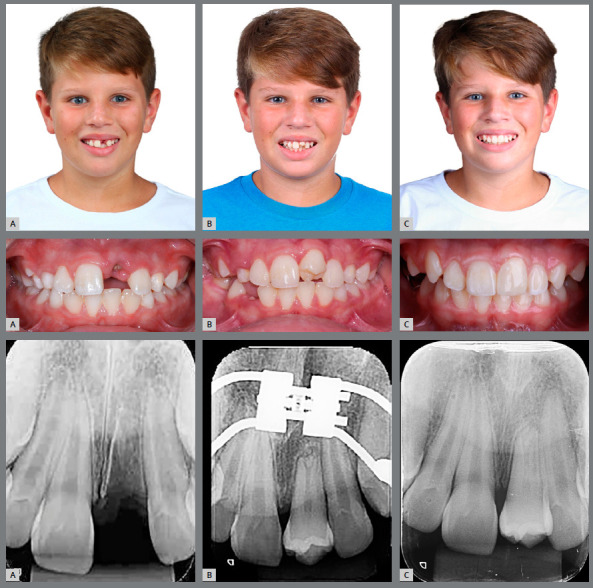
Photographic and radiographic control: A) initial; B) post-surgical; C) post-restoration.
